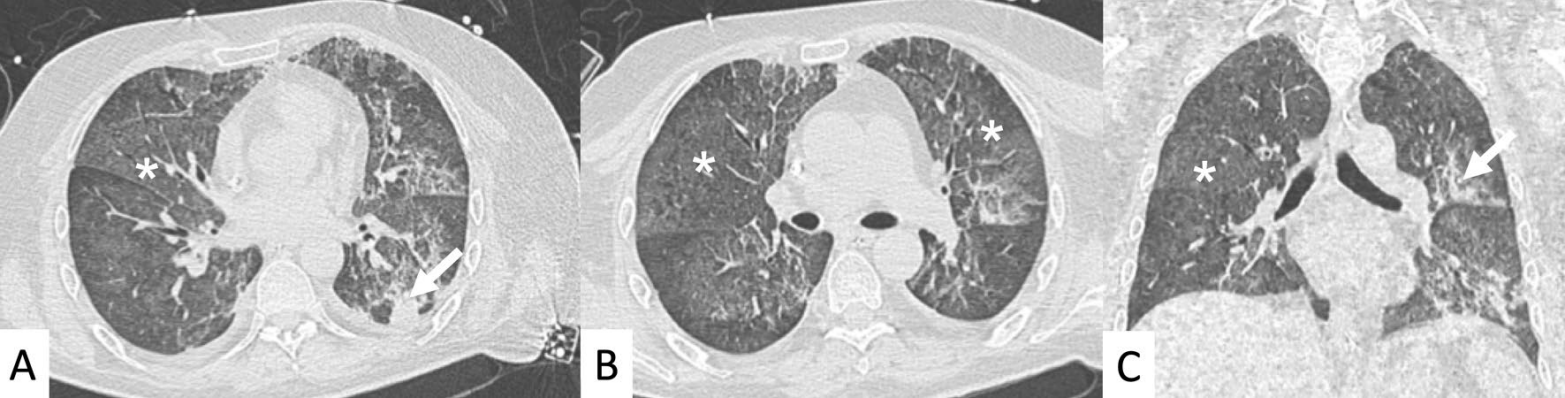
A 62-year-old man with acute respiratory failure. He referred Gottron papules and acral cutaneous ulcerations one month previously; laboratory exams revealed increased Creatine-Phosphokinase levels (800 u/l) and presence of Anti-melanoma differentiation-associated gene 5 (MDA5) antibodies. Diffuse ground-glass opacifications (white asterisks in A–C) and small consolidations (white arrows in A, C) are depicted, suggesting HRCT features of diffuse alveolar damage
